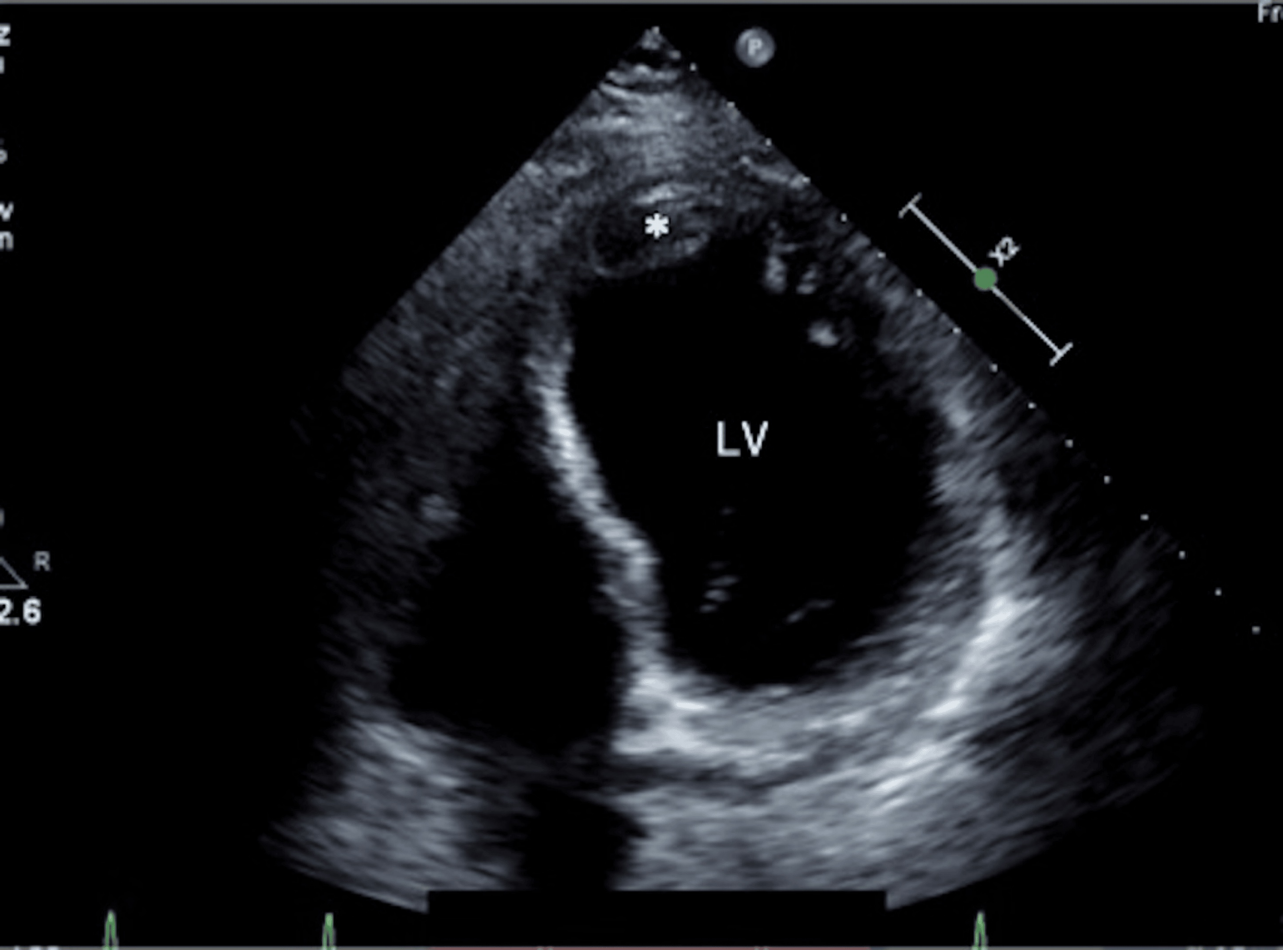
Transthoracic echocardiography showing a thrombus (*) at the left ventricle LV: left ventricle
